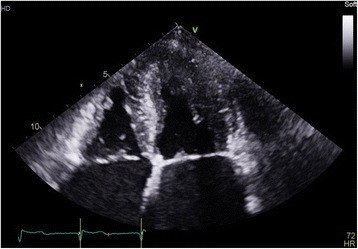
A different view of apical four-chamber echocardiography showing apical left ventricular hypertrophy
